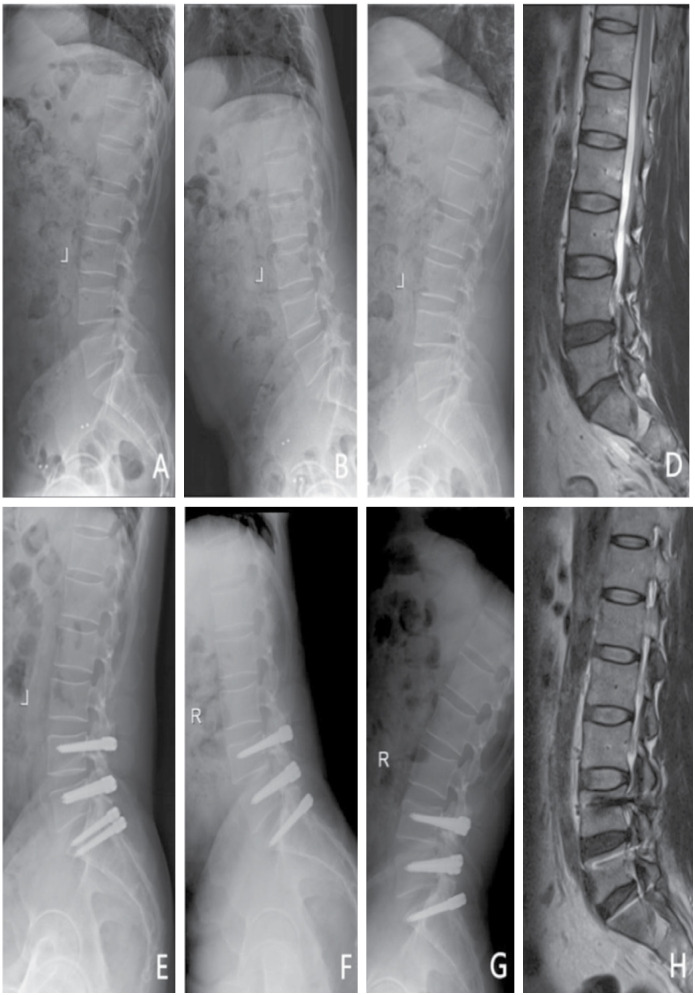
A 47-year-old male patient underwent surgery with Dynesys system due to lumbar disc herniation and spinal stenosis in L4/5 and L5/S1. (A) Pre-operation lateral X-ray. (B–C) Pre-operation flexion and extension X-ray, the ROM of operating segments was 6.5°, and that of the upper adjacent segment was 1.7°. (D) Pre-operation T2WI MRI demonstrated L4/5 and L5/S1 disc herniation. E: Lateral X-ray at 32 months after surgery. (F–G) Flexion-extension X-ray at 32 months after surgery, the ROM of operating segments was 1.7°, and that of the upper adjacent segment was 4.7°. (H) T2WI MRI at 32 months after surgery.
